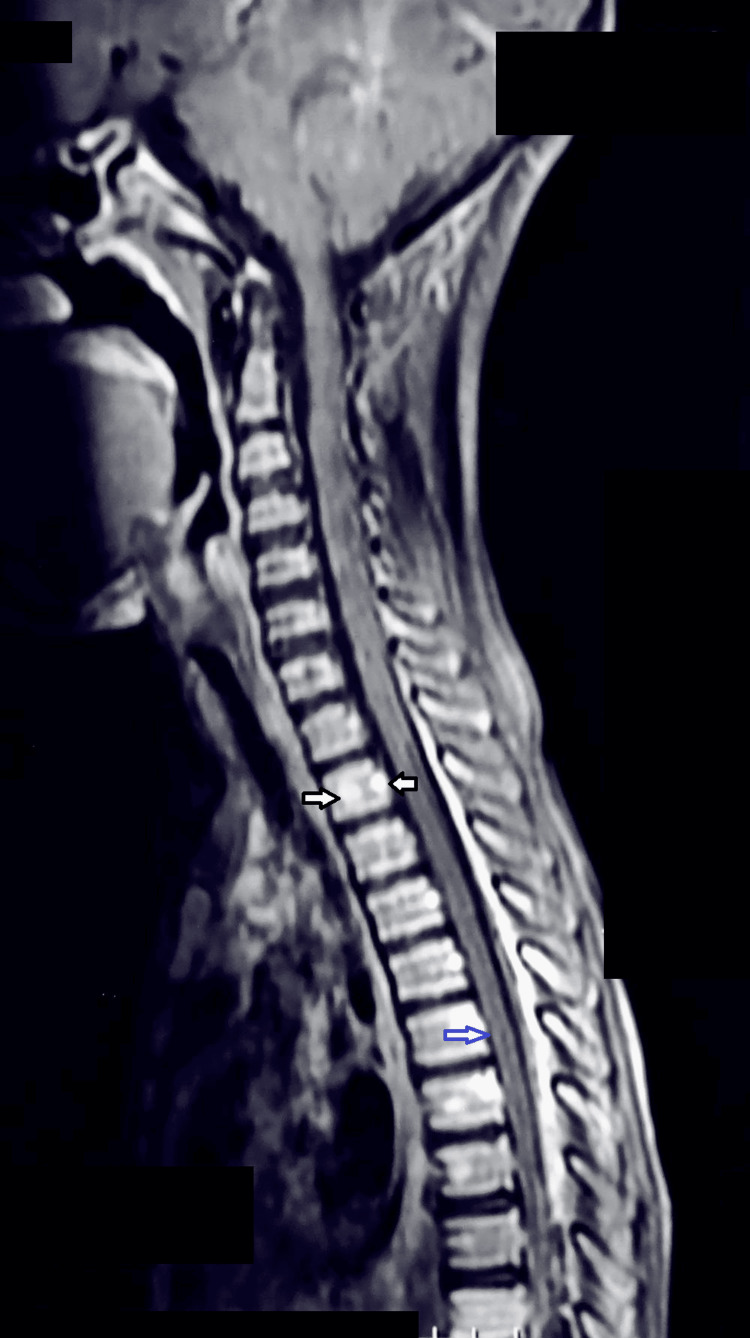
Sagittal T1-weighted MRI of the spine showing vertebral involvement (white arrows) and associated spinal cord compression (blue arrow) MRI: magnetic resonance imaging
